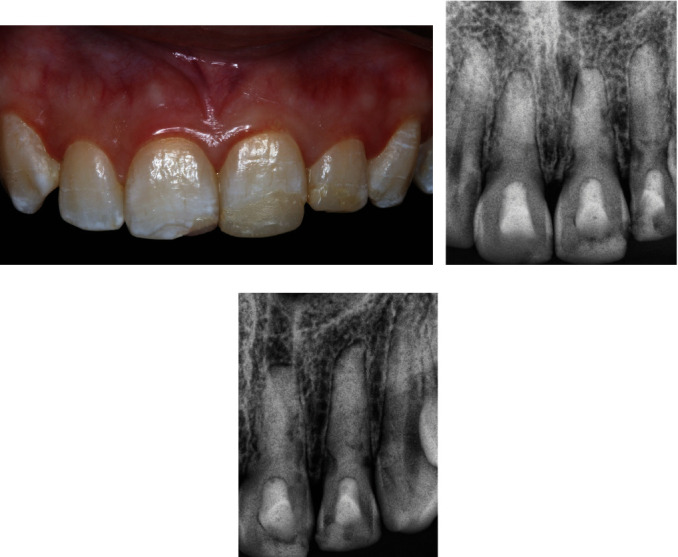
Clinical and radiographic appearance at the 60-month follow-up. (a) Intraoral frontal view of the patient. (b) and (c) Follow-up radiographs.
